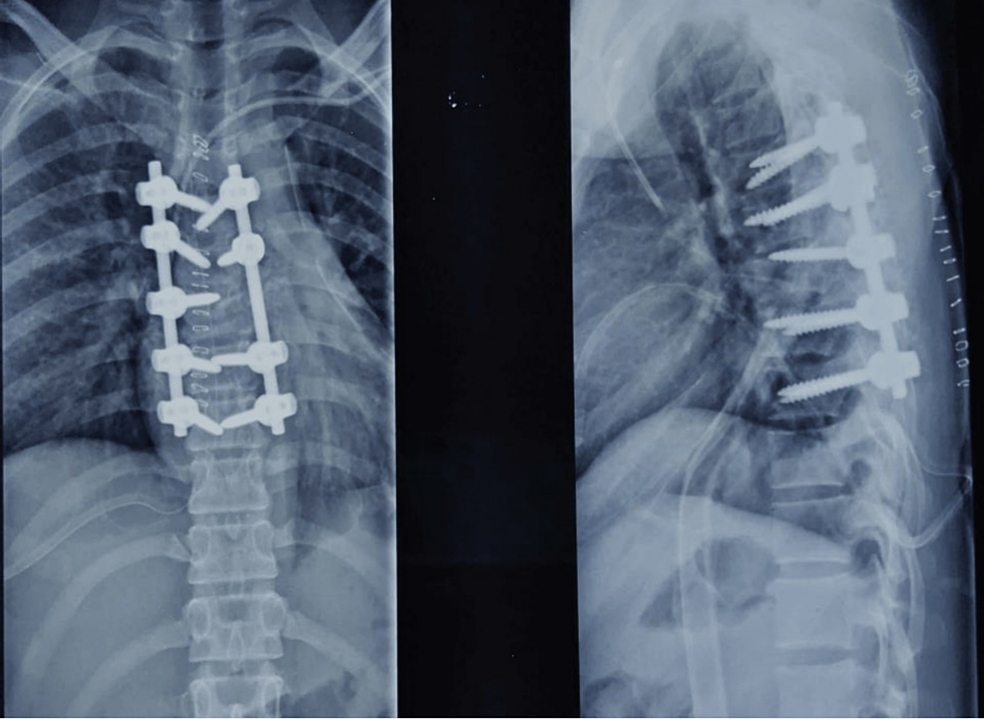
Postoperative X-ray after fixation – AP and lateral view AP, anteroposterior.
